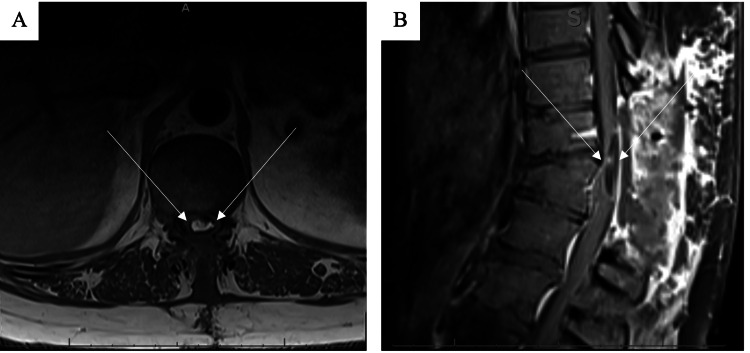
Post-operative MRI of the spine A) Post-operative T1 sagittal MRI of the thoracic cord showed a residual 3.8 cm x 1.1 cm x 0.6 cm lesion at the level of T11. B) Post-operative T1 axial MRI shows moderate to severe central canal stenosis and mass effect, improved from prior examination.
